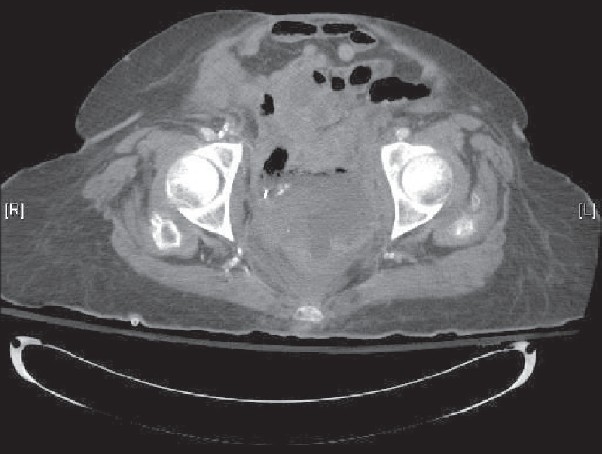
Abdominal CT scan showing bladder infiltration from the squamous cell carcinoma of the rectum
